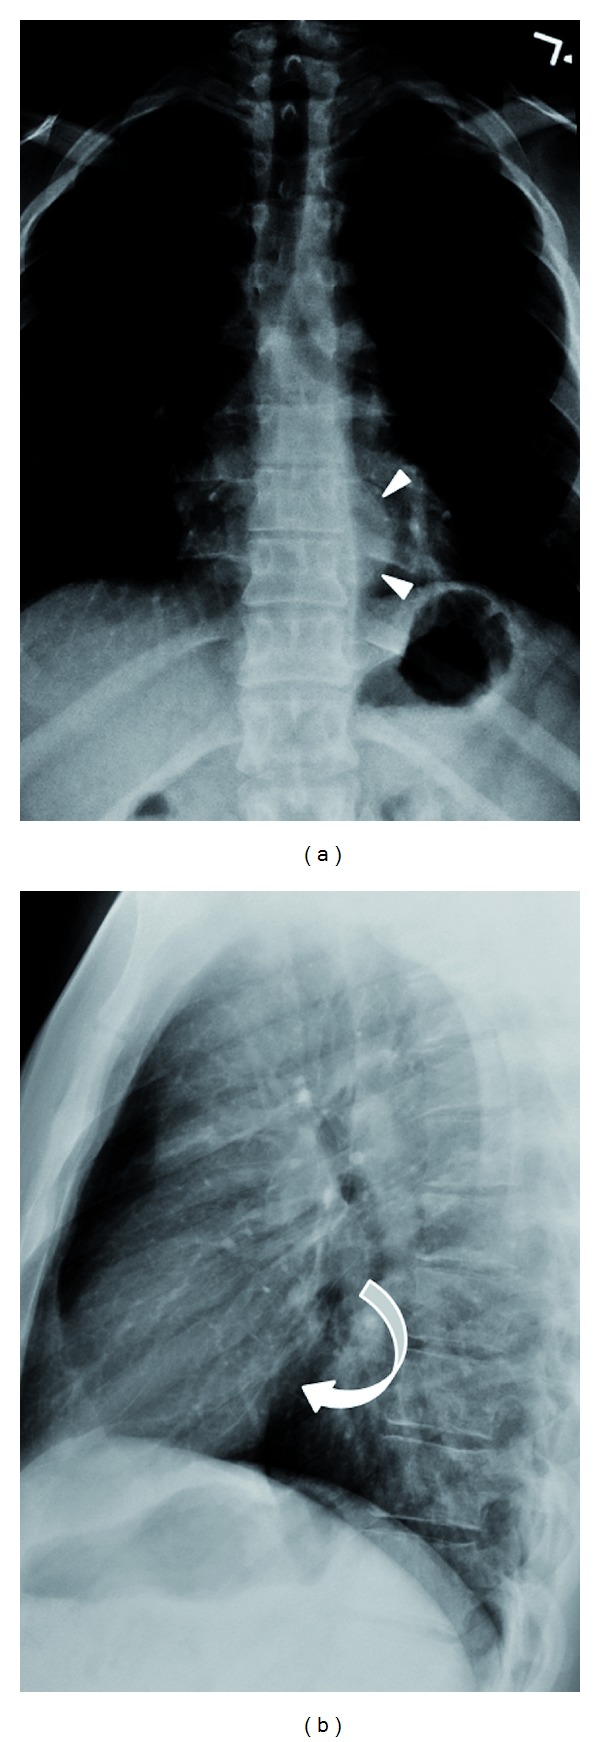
(a) Frontal radiograph shows the presence of a hemispheric, retrocardiac opacity superior to the left cardiophrenic sulcus (arrowheads). (b) Lateral radiograph demonstrates a subtle rounded lucency marginating the posterior aspect of the heart (curved arrow).
